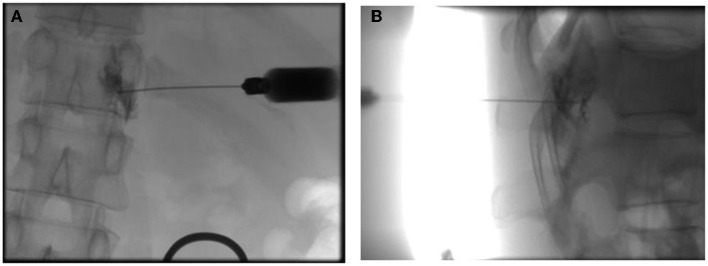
Confirmation of the extent of the block with contrast under the C-arm X-ray machine positioning. (A) Anteroposterior film showing the needle tip's position at the connecting line of the intervertebral foramen on the affected side and the entry of the contrast agent into the spinal canal along the nerve root. (B) Lateral film of the upper half of the intervertebral foramen on the affected side.
